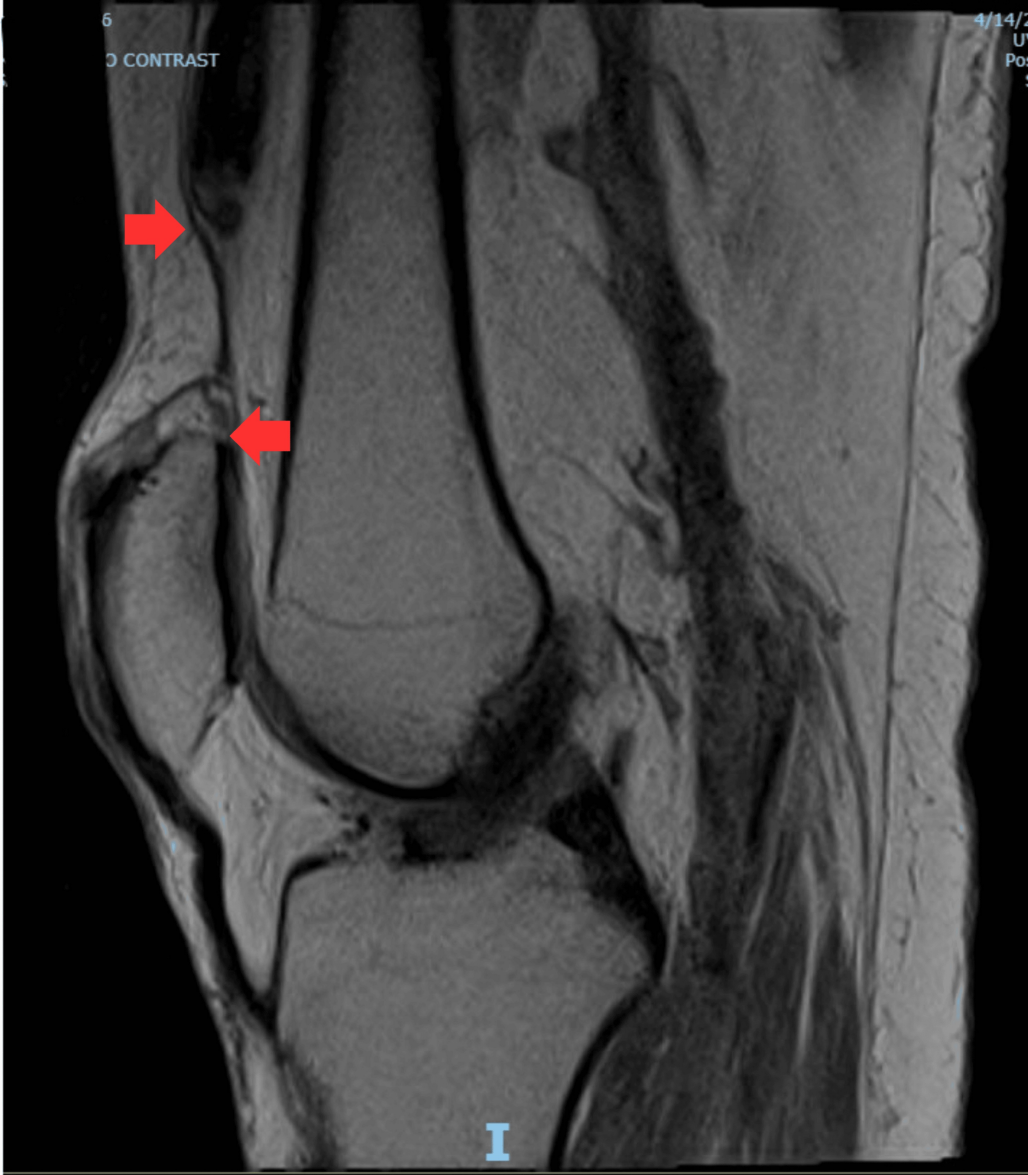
Right Knee MRI, Lateral View Lateral view of right knee MRI (T1) performed at physiatrist’s recommendation demonstrating a “complete tear of the distal quadriceps tendon attachment with 2.7cm of retraction,” felt possibly subacute due to fluid in the region. The red arrows indicate the void left by QT retraction. QT: Quadriceps tendon
